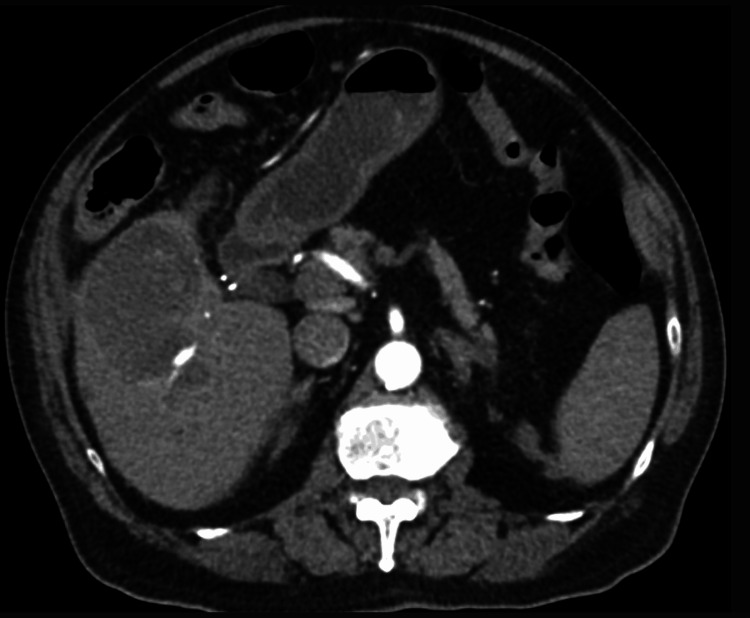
A CT scan of the abdomen showing a mycotic aneurysm in the posterior branch of the right hepatic artery within a liver abscess.
